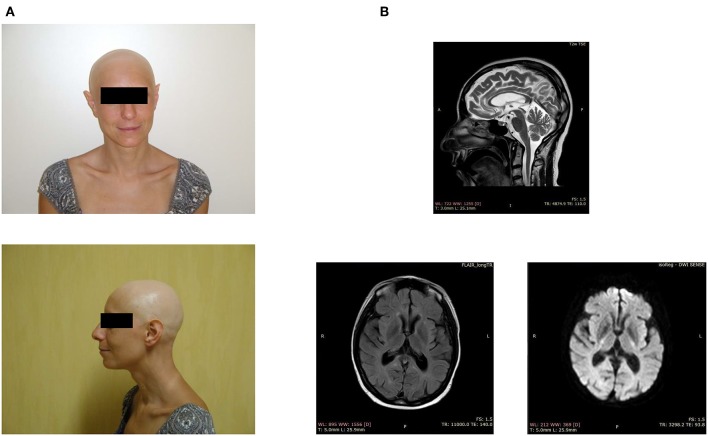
Clinical features of the index case. (A) Facial appearance of the patient, showing alopecia and sharp ears. (B) Brain MRI demonstrates periventricular white matter hyperintensities, ventriculomegaly, and a thin corpus callosum.
